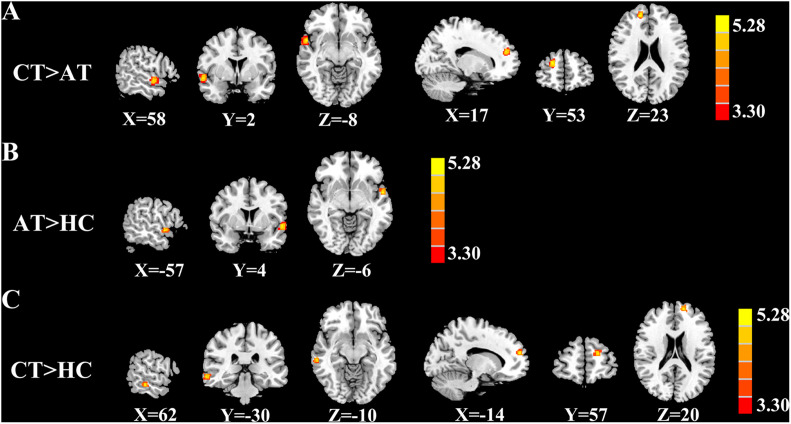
Significant CBF differences among the chronic tinnitus (CT), acute tinnitus (AT), and healthy controls (HC). (A) Compared to AT, CT showed increased z-CBF in the right superior temporal gyrus (STG), and right superior frontal gyrus (SFG). (B) Compared to HC, AT showed higher z-CBF in the left STG. (C) Compared to HC, CT showed increased z-CBF in right middle temporal gyrus (MTG) and left SFG. Significant thresholds were corrected using FDR criterion and set at *p* < 0.01.
